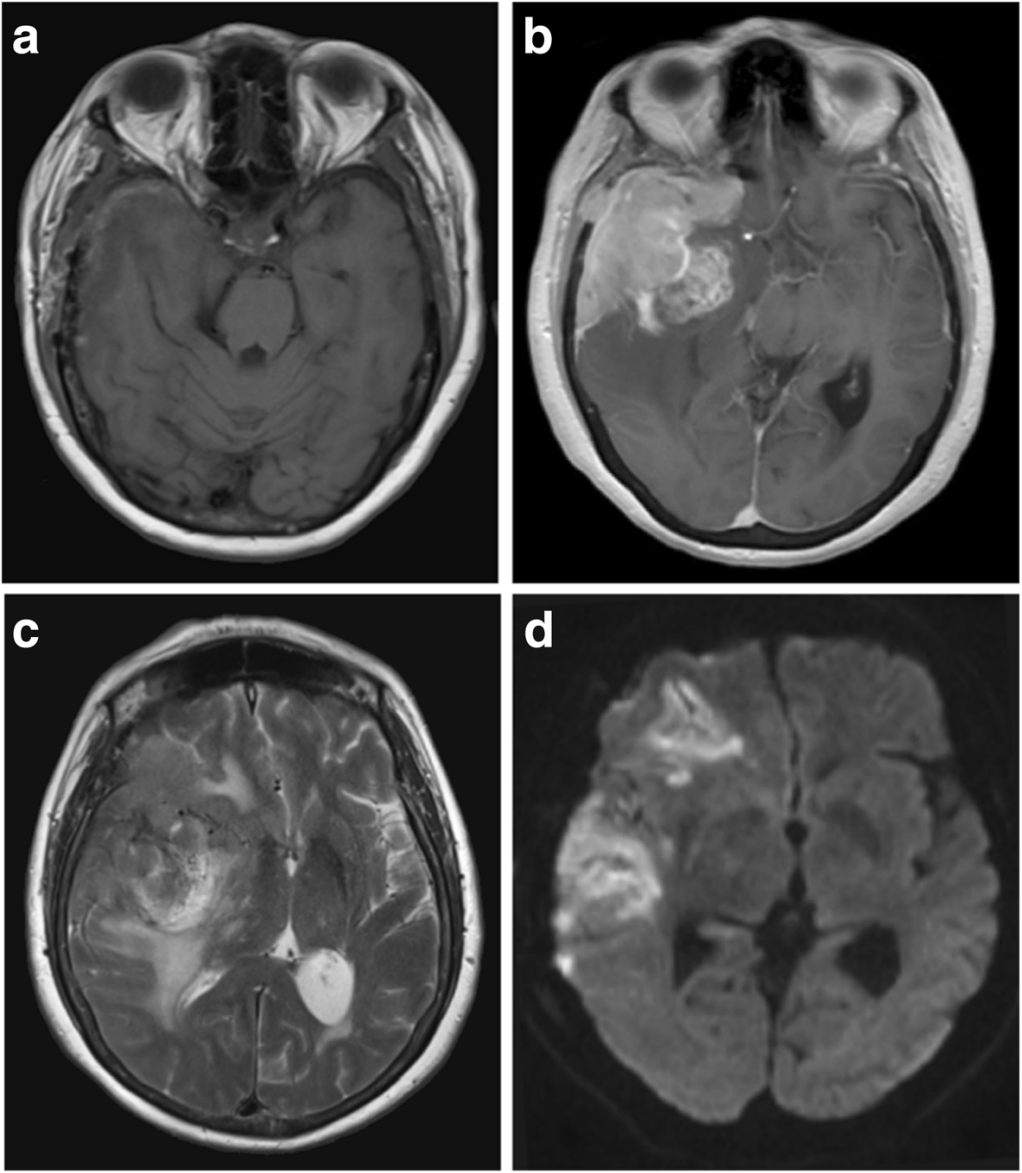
Atypical meningioma. a, b Pre- and post-contrast axial T1-weighted MR images showing a heterogeneously enhancing mass with a wide dural bass, dural tail and CSF cleft. The cortical interface is poorly outlined suggestive invasion of the underlying brain parenchyma as well as the adjacent skull. c Axial T2-weighted MR image of the same lesion with surrounding vasogenic oedema and midline shift. d A diffusion-weighted image shows heterogeneous areas of restricted diffusion extending into the adjacent cortex
